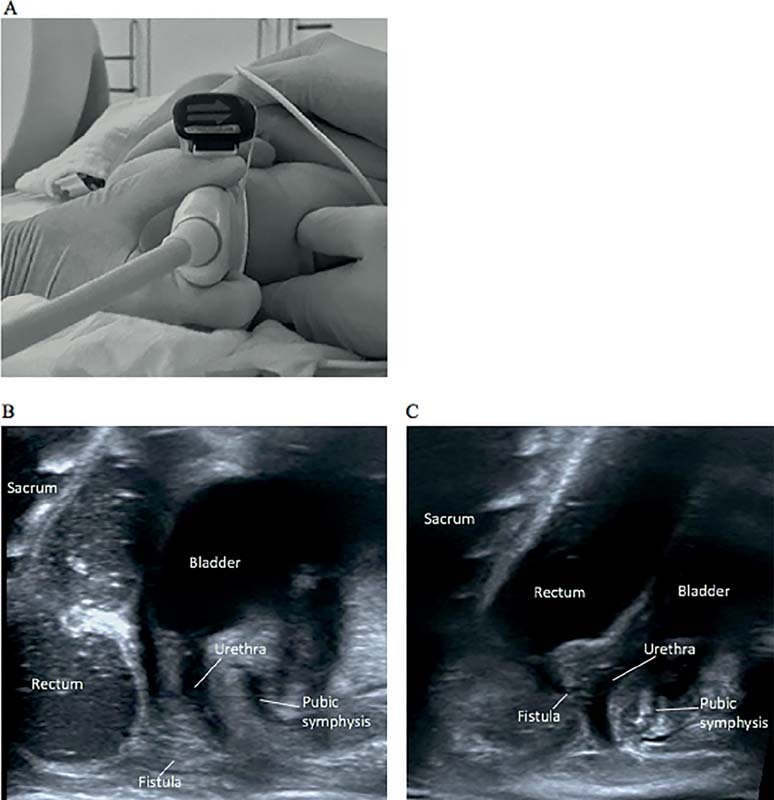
High resolution perineal ultrasound images: position of the linear transducer with PIUR tUS Infinity System add on mediansagittal at the center of the perineal body, patient supine held in lithotomy position, foley cathter in the mucous fistula. (
